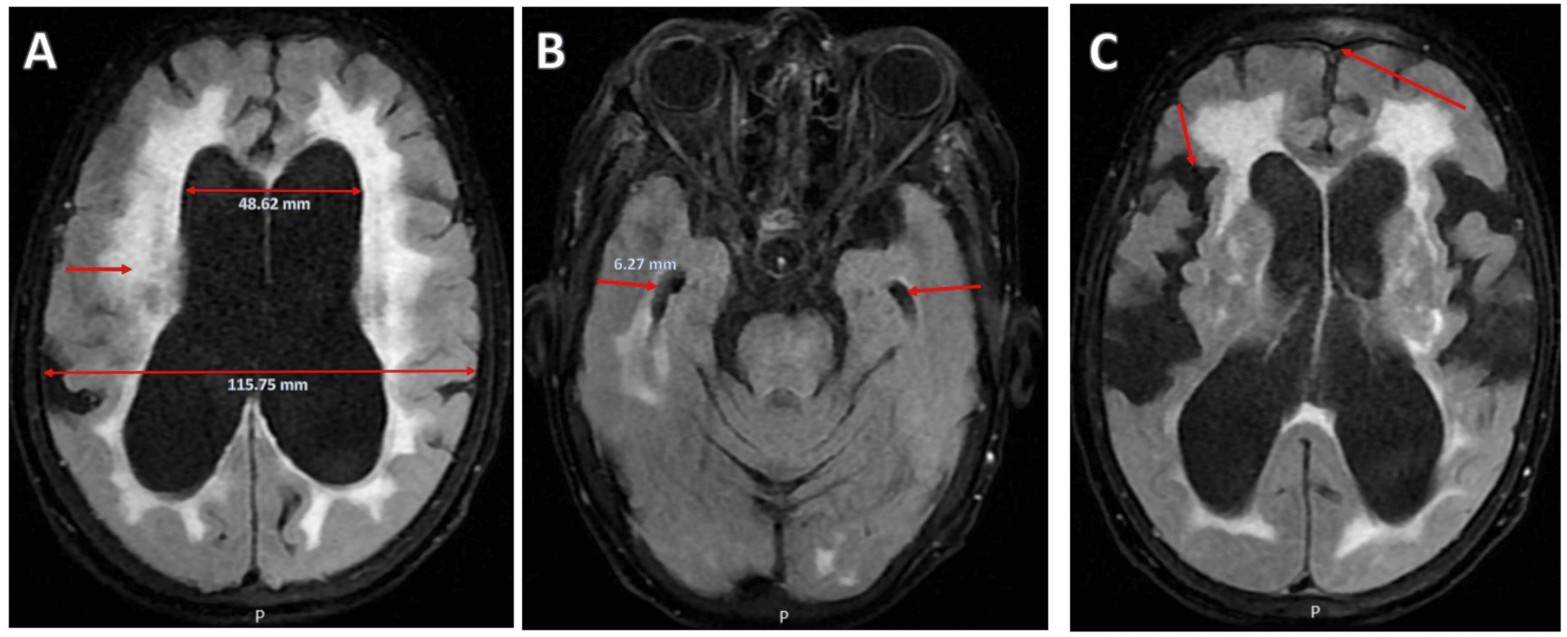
Brain MRI FLAIR sequences showing findings consistent with iNPH A: Axial FLAIR image demonstrating ventriculomegaly with increased transverse diameter of the lateral ventricles (double-headed arrows). Note the presence of symmetric periventricular hyperintensities adjacent to the ventricular margins (single arrow), consistent with transependymal CSF seepage. B: Axial FLAIR image at the level of the temporal lobes shows prominent temporal horns (arrows), a hallmark of ventricular dilation in iNPH. C: Axial FLAIR image highlighting DESH pattern, characterized by narrowed high-convexity sulci (long arrow) and enlarged Sylvian fissures (short arrow), supporting the diagnosis of iNPH. MRI: magnetic resonance imaging, FLAIR: fluid-attenuated inversion recovery, iNPH: idiopathic normal pressure hydrocephalus, CSF: cerebrospinal fluid, DESH: disproportionately enlarged subarachnoid space hydrocephalus
